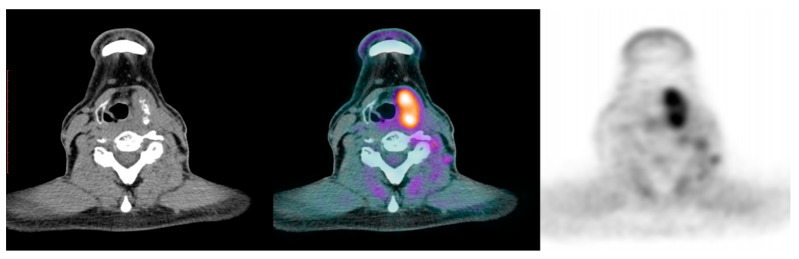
Increased 18-F FDG uptake in the left sphenoid sinus. First image: CT scan, second image: merged CT and PET scan, third image: FDG uptake.
